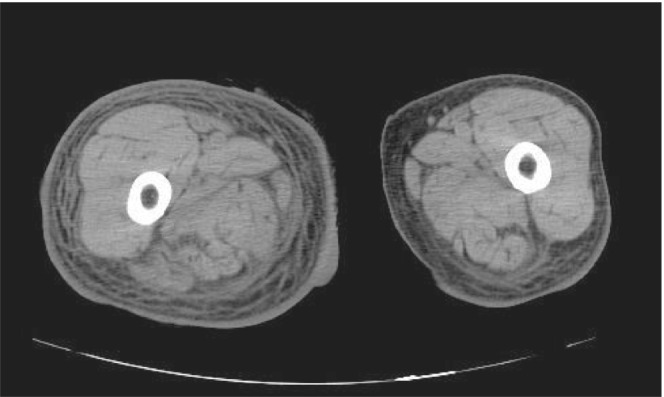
CT scan of the thighs. CT scan of the thighs showed evidence of increased fatty tissue density all around the right thigh.
